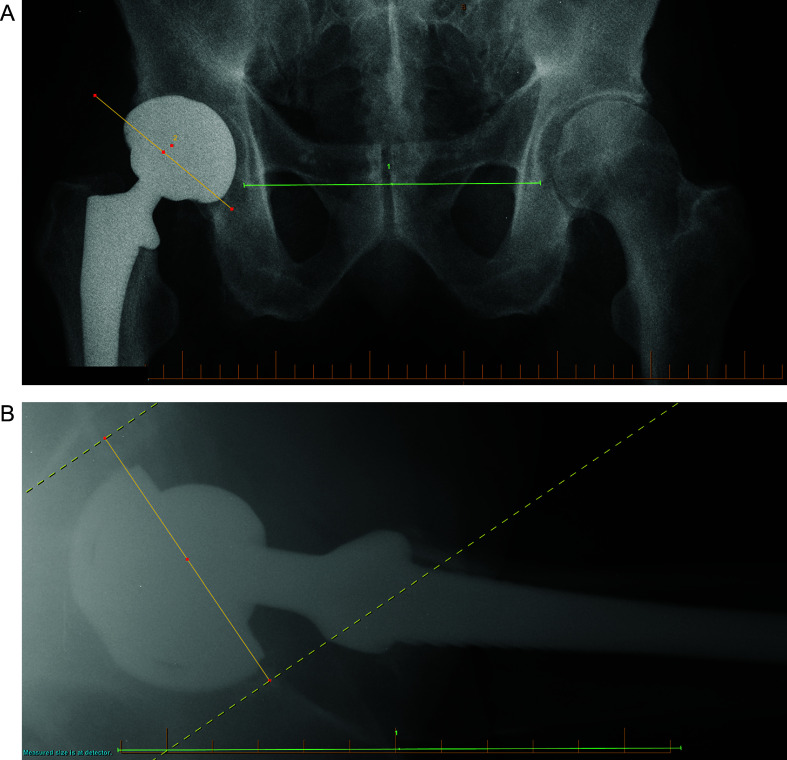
Acetabular cup inclination was determined as the angle (in degrees) between a line drawn along the rim of the cup and the teardrop line on the AP pelvis (A). Acetabular cup anteversion was measured on the cross-table lateral radiograph as the angle (in degrees) between a line drawn along the rim of the cup and a line perpendicular to the horizontal plane (B).
